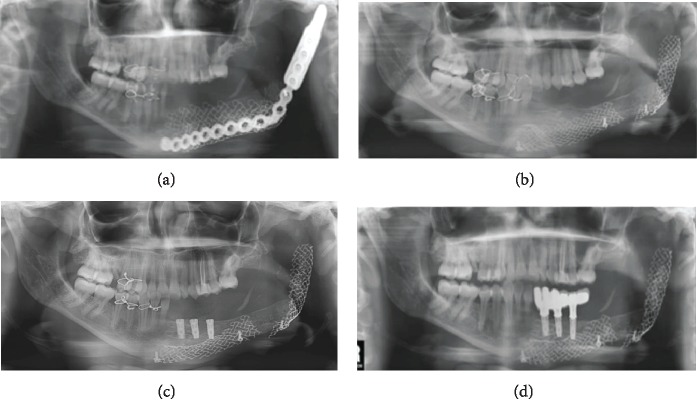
(a) Orthopantomogram (OPG) at the three-month follow-up. (b) OPG after removing the reconstruction plate. Bone regeneration can be observed on the left mandible. (c) OPG showing implant rehabilitation. (d) OPG showing prosthetic rehabilitation with dental implants and an implant-supported partial denture.
